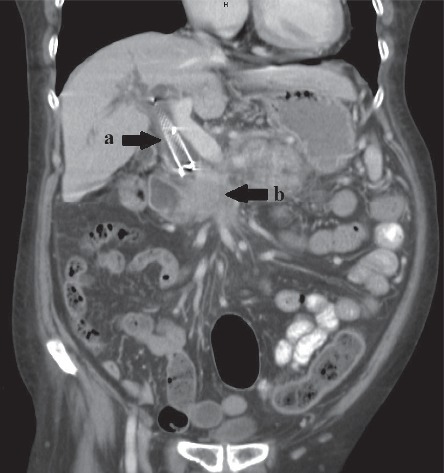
Computed tomography scan performed after the patient developed recurrent obstructive jaundice showing proximal migration of the previously placed metallic stent (a). The distal end of the stent was located above the pancreatic mass (b), and upstream dilatation of the common bile duct was apparent.
